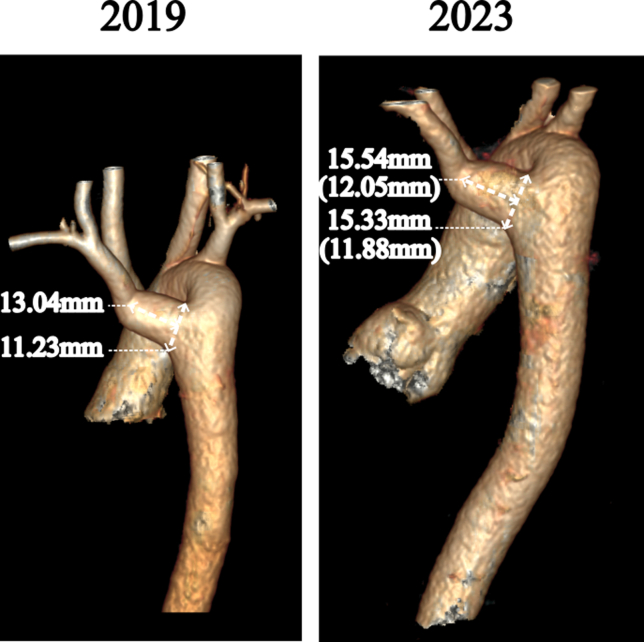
Reconstructions of patient aorta from computed tomography.
